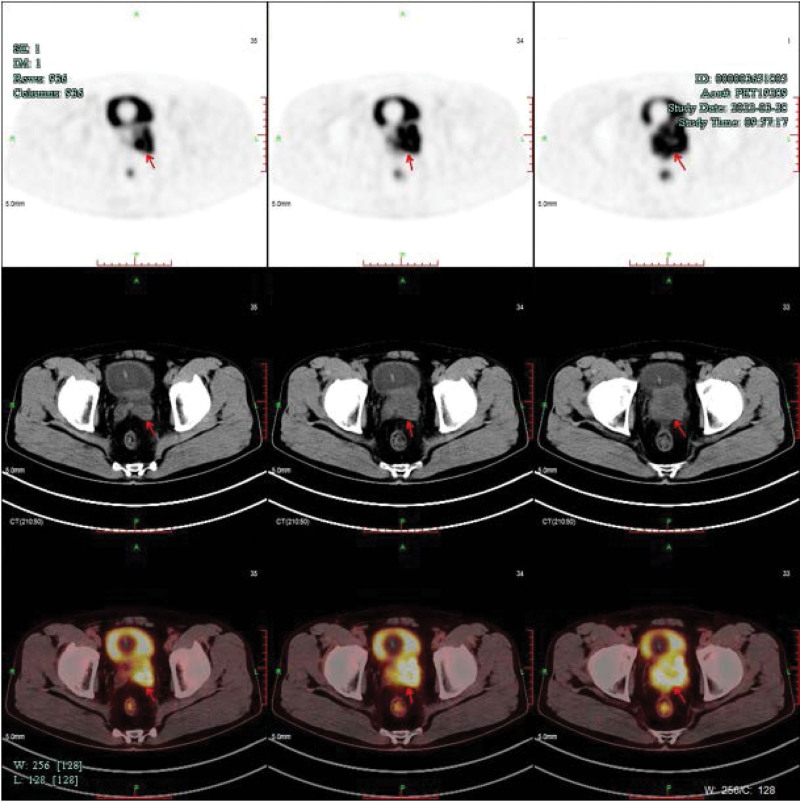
PET-CT of prostate disease at baseline on 2022-03-28. PET-CT = positron emission tomography-computed tomography.
